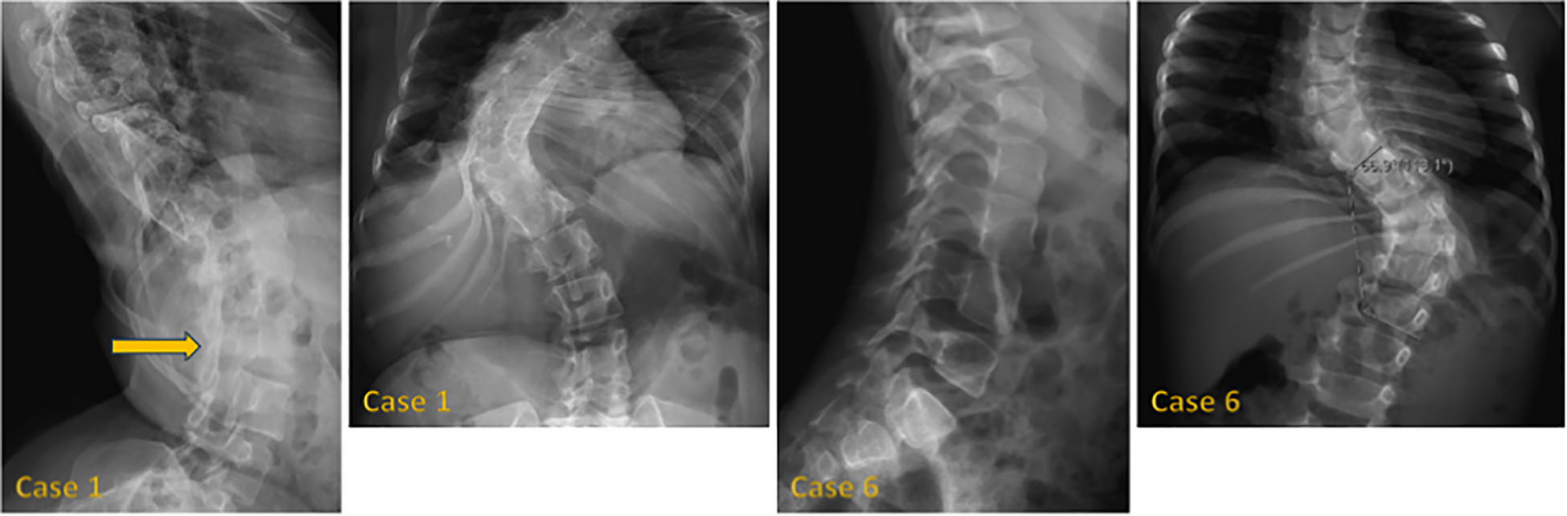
Lateral radiographs of case 1 reveal lumbar hyperlordosis (arrow), while frontal radiographs show thoracolumbar scoliosis and abnormal rib morphology. In case 6, lateral radiographs demonstrate tall lumbar vertebral bodies, and frontal radiographs display thoracolumbar scoliosis.
